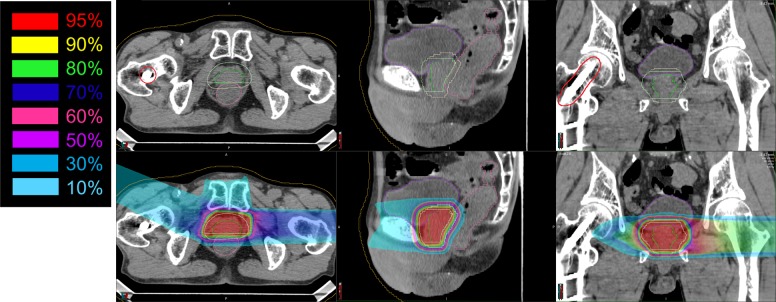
Clinical case data of a patient with a metal implant. The upper row shows CT images and the bottom row shows CT images together with the dose distribution. Left column shows axial images, middle column shows sagittal images, and right column shows coronal images. Red lines show the metal implant after hip replacement. Green line shows prostate, light yellow line shows PTV, magenta line shows rectum, and purple line shows the bladder.
